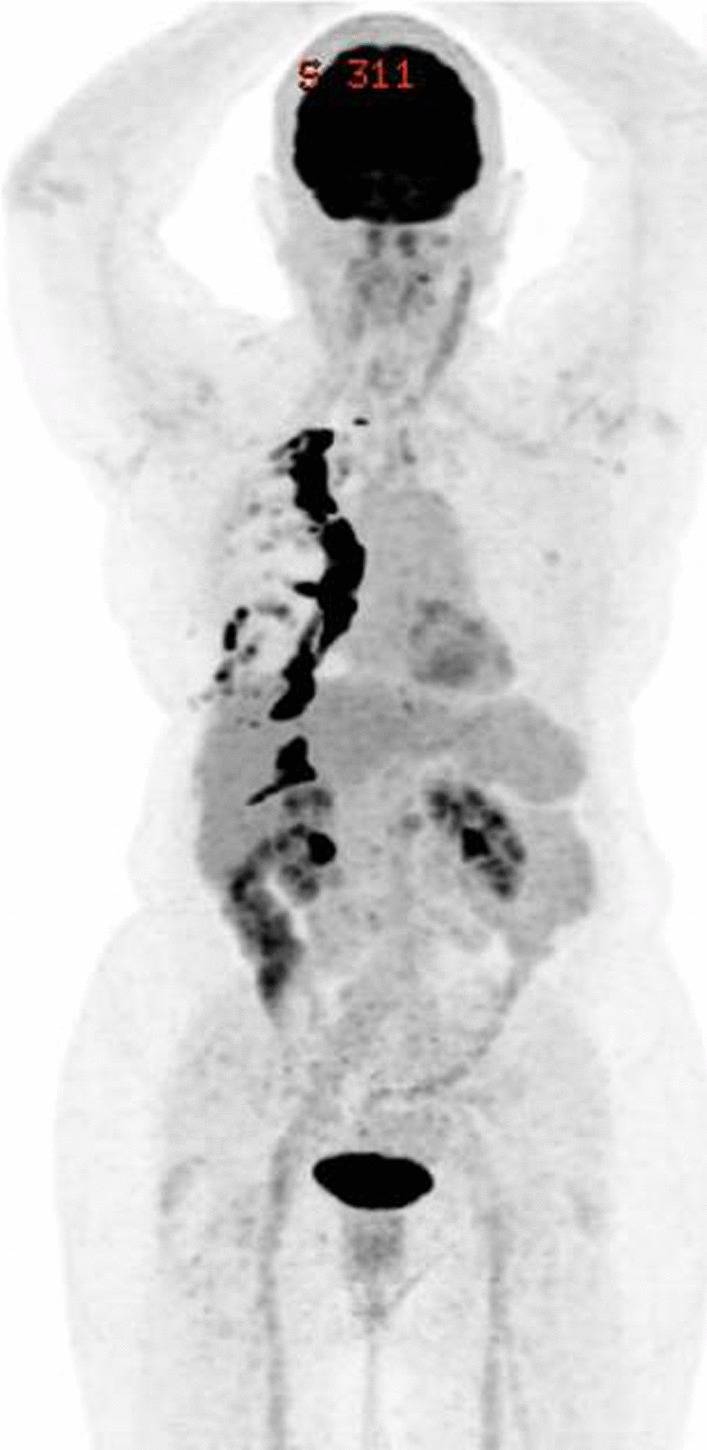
FDG-PET scan showing no suspicious FDG uptake within lungs. There is no scan evidence of pericardial effusion. The foci of intense FDG uptake surrounding right lung pleura are consistent with a previous talc pleurodesis
